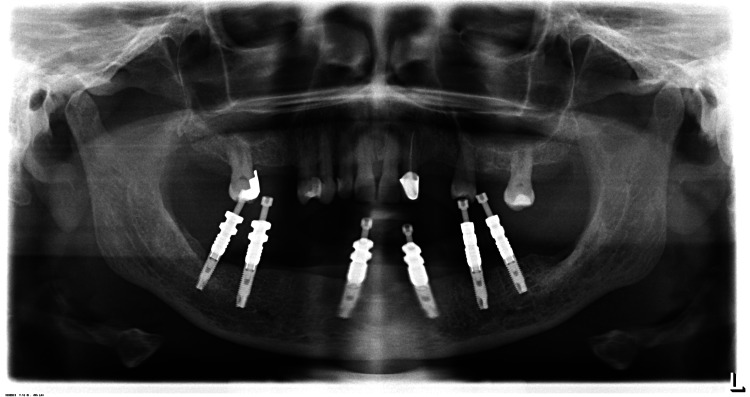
Panoramic radiograph to verify complete seating of impression posts
